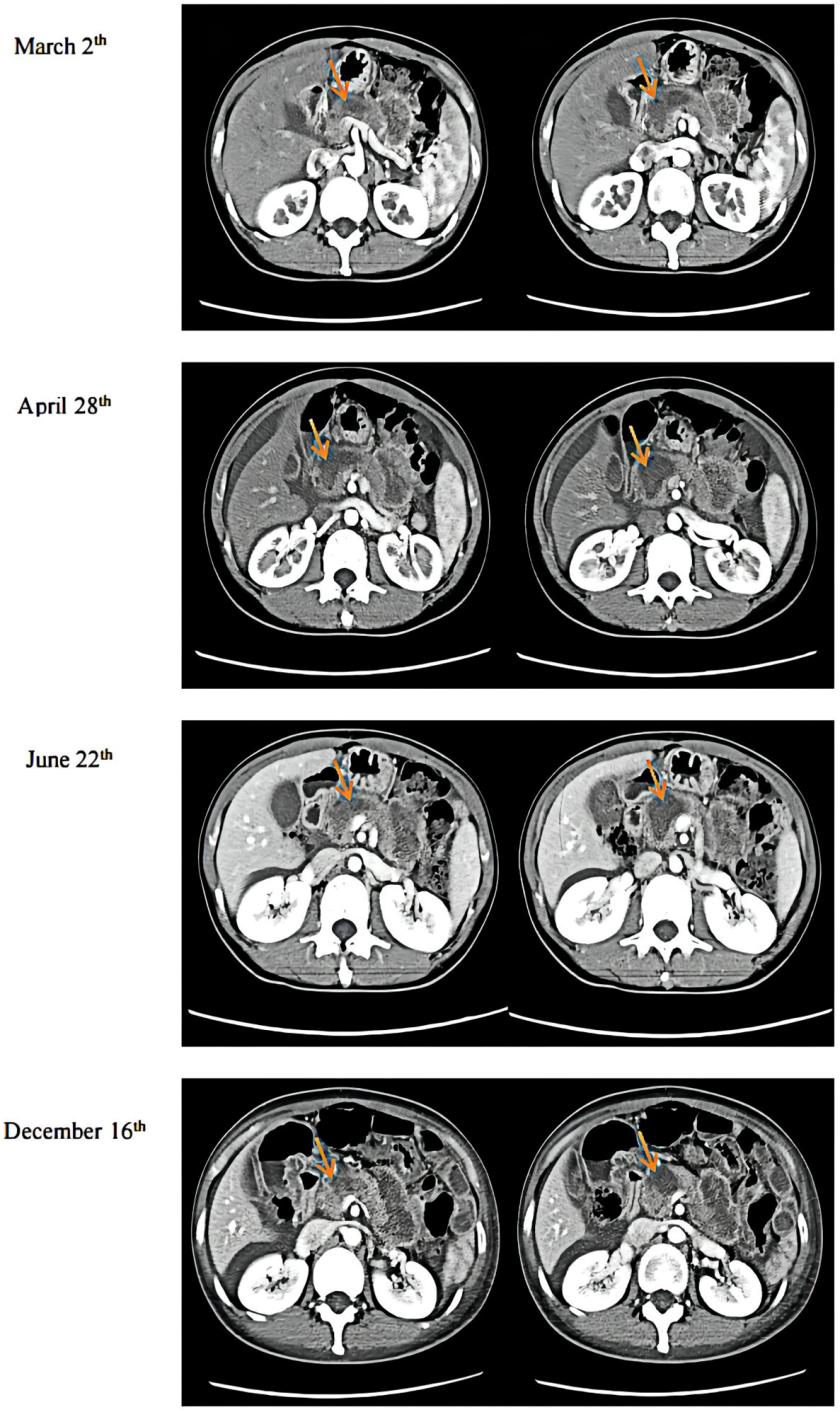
CT examination results. March 2^nd^ was during anlotinib treatment. April 28^th^ was before radiotherapy. June 22^nd^ was after immunotherapy plus radiotherapy, and December 16^th^ was during maintenance immunotherapy.
